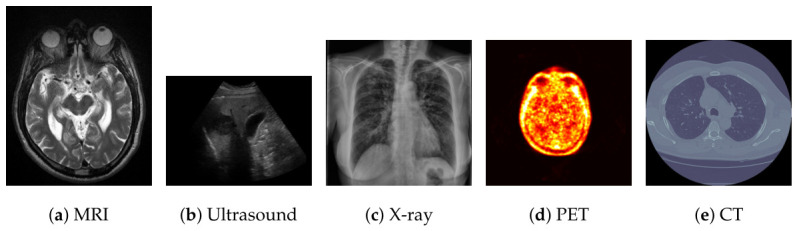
Representative examples of medical imaging modalities commonly used in precision oncology and radiomics: (a) Magnetic Resonance Imaging (MRI), (b) Ultrasound (US), (c) Chest X-ray (CXR), (d) Positron Emission Tomography (PET), and (e) Computed Tomography (CT). Representative images were obtained from publicly available datasets [3,4,5,6,7].
